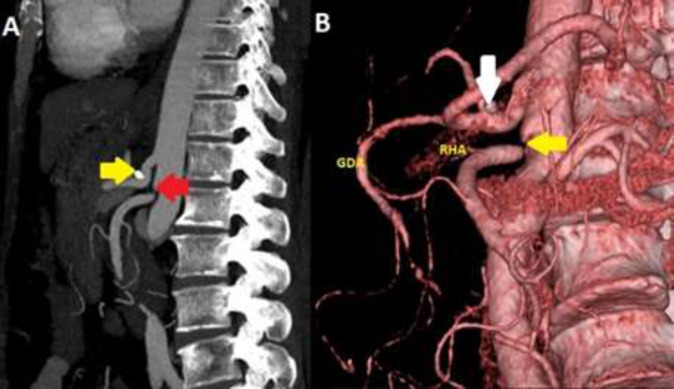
A: Postoperative computed tomography angiography shows that celiac artery compression improves, but superior mesenteric artery stenosis continues (red arrow). In addition, the clip placed in the phrenic artery is seen as hyperdense (yellow arrow). B: In the three dimensional computed tomography angiography, collateral circulation is observed between the stenotic superior mesenteric artery (yellow arrow) and the celiac artery through the gastroduodenal artery (GDA). The right hepatic artery (RHA) originates from the superior mesenteric artery. A metallic clip can be seen adjacent to the noncompressed celiac artery root (white arrow)
